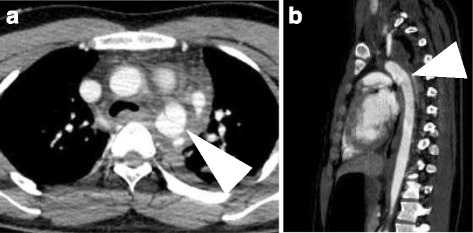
Preoperative contrast-enhanced computed tomography. a Horizontal dislocation. b Sagittal section. The arrowhead shows dissection in zones 3 to 4 of the thoracic aorta
